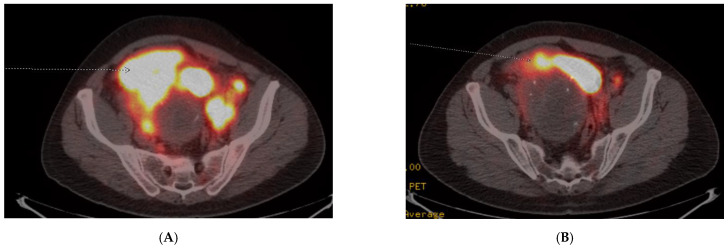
Case 3, (A) Baseline Gallium-68 Dotatate PET-CT demonstrated a large pelvic mass with retroperitoneal adenopathy and ipsilateral ureteric compression; (B) Follow-up Gallium-68 Dotatate PET-CT after 3 cycles of Lu-177 Dotatate demonstrated significant reduction in pelvic mass and retroperitoneal adenopathy.
